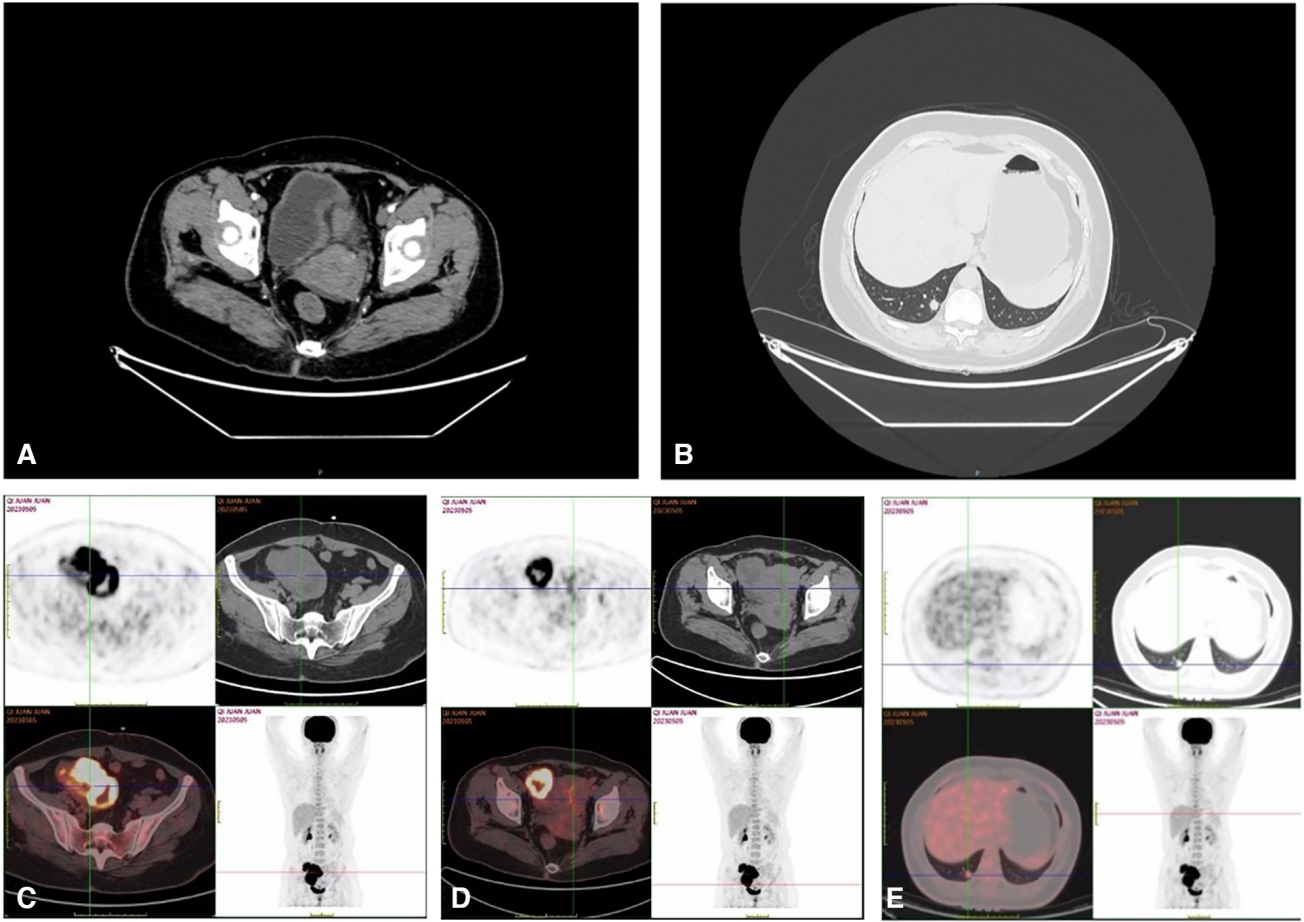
Pelvic CT showed huge mass in the right adnexal area (A); chest (lung) CT showed nodule in the lower lobe of the right lung (B); ^18^F-FDG PET-CT showed that the right pelvic cystic solid mass and the mass uptake of FDG increased obviously, SUVmax = 22.8 (C,D); ^18^F-FDG PET-CT showed that the hypermetabolic nodule in lower lobe of right lung, SUVmax = 3.8 (E).
